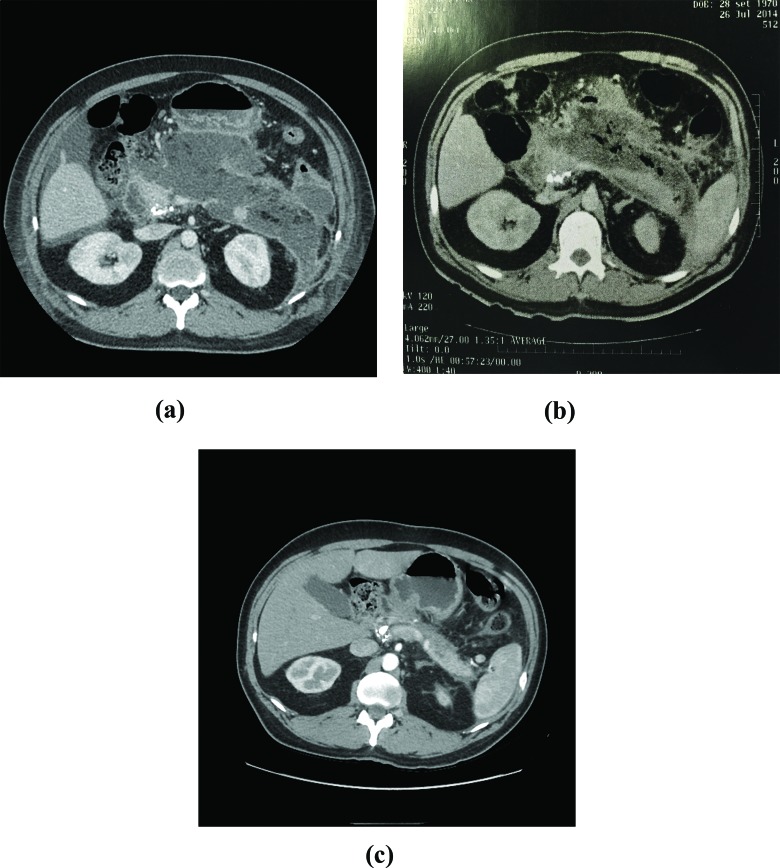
CT scan, case 5. A: First exam, 10 days after the onset of symptoms: extensive necrosis of the body and tail of the pancreas; calcification in the pancreatic head, suggesting the presence of intraductal neoplasia. B: After 30 days of follow-up (increase in CRP and fever): gas in the full extent of the area of pancreatic necrosis. C: 5 months after hospital discharge: no pancreatic necrosis or collection.
